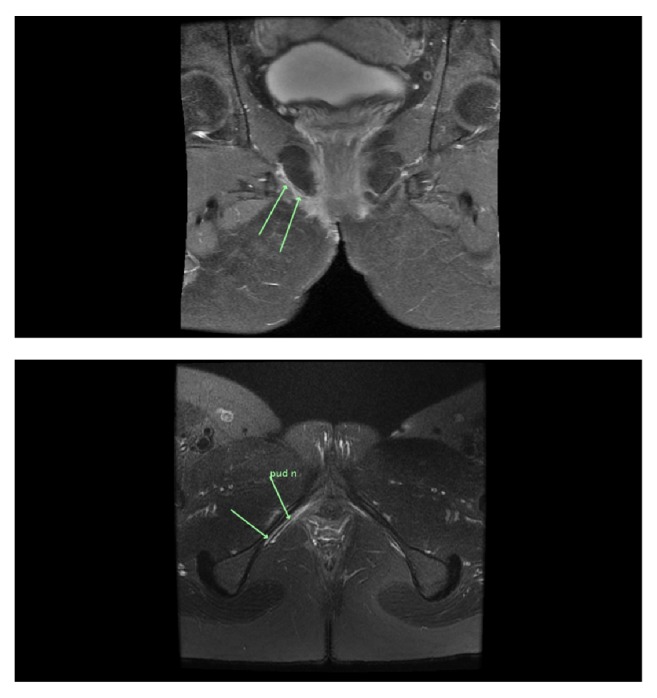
Postoperative T2 axial MRI through the pelvis demonstrating high T2 signal in the right pudendal nerve.
